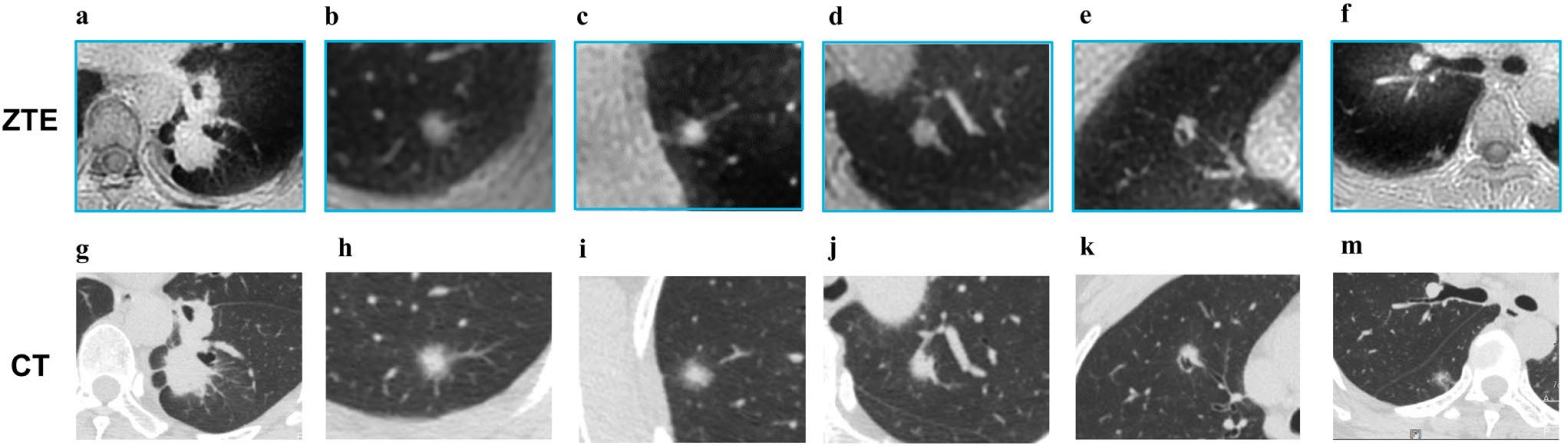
Female, 49 years old, multiple nodules in both lungs, dorsal roundish mass in the upper lobe of the left lung with clear borders and hairy edges, with lobulation, tracheal truncation, and cavity formation. (a–f) ZTE shows multiple nodules; (g–m) CT images correspond to ZTE.
